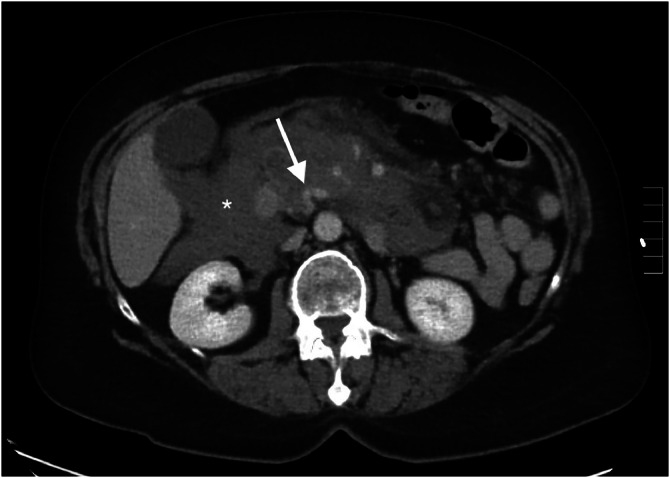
Portal venous phase CT of the abdomen and pelvis reveals acute mesenteric hemorrhage (asterisk) with formation of prominent, serpiginous splanchnic collateral vessels (arrow).
